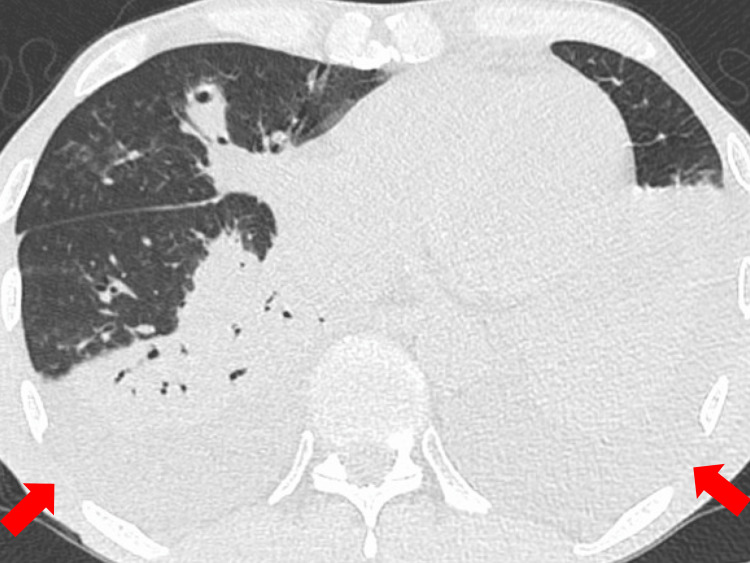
Chest computed tomography imaging on the eighth day of admission Chest computed tomography showing bilateral pleural effusions, despite a reduction in the infiltrating shadows
